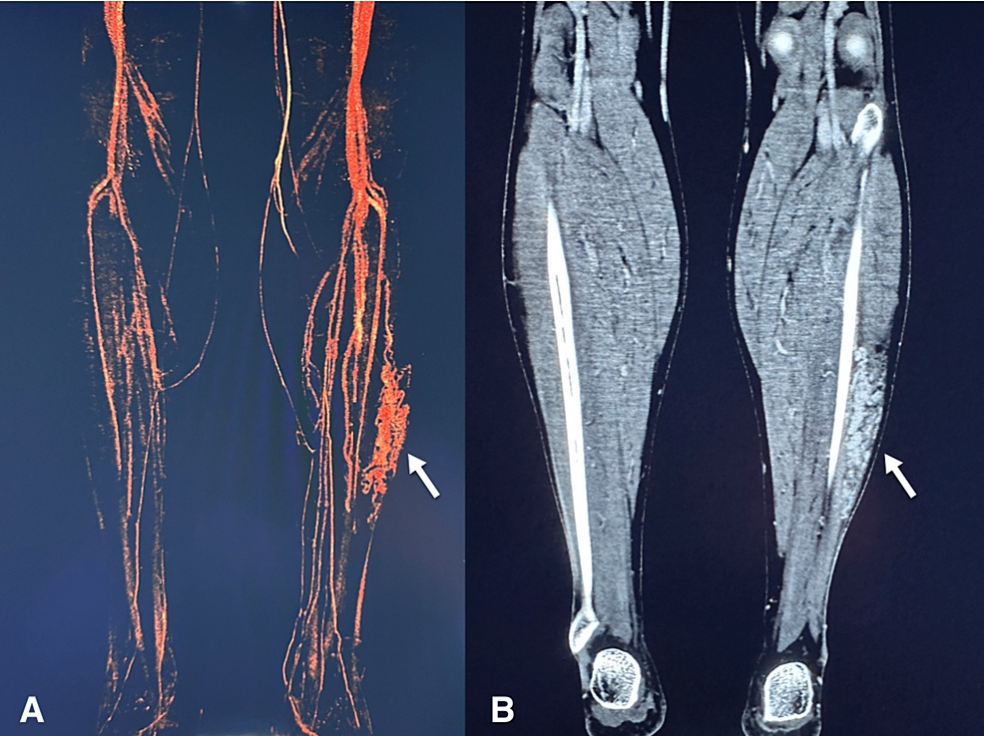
CTA maximum intensity projection rendering of the lower-extremity vasculature shows an intramuscular arteriovenous malformation (arrows) of the lateral left calf with evidence of an early venous return. CTA, computed tomography angiography
